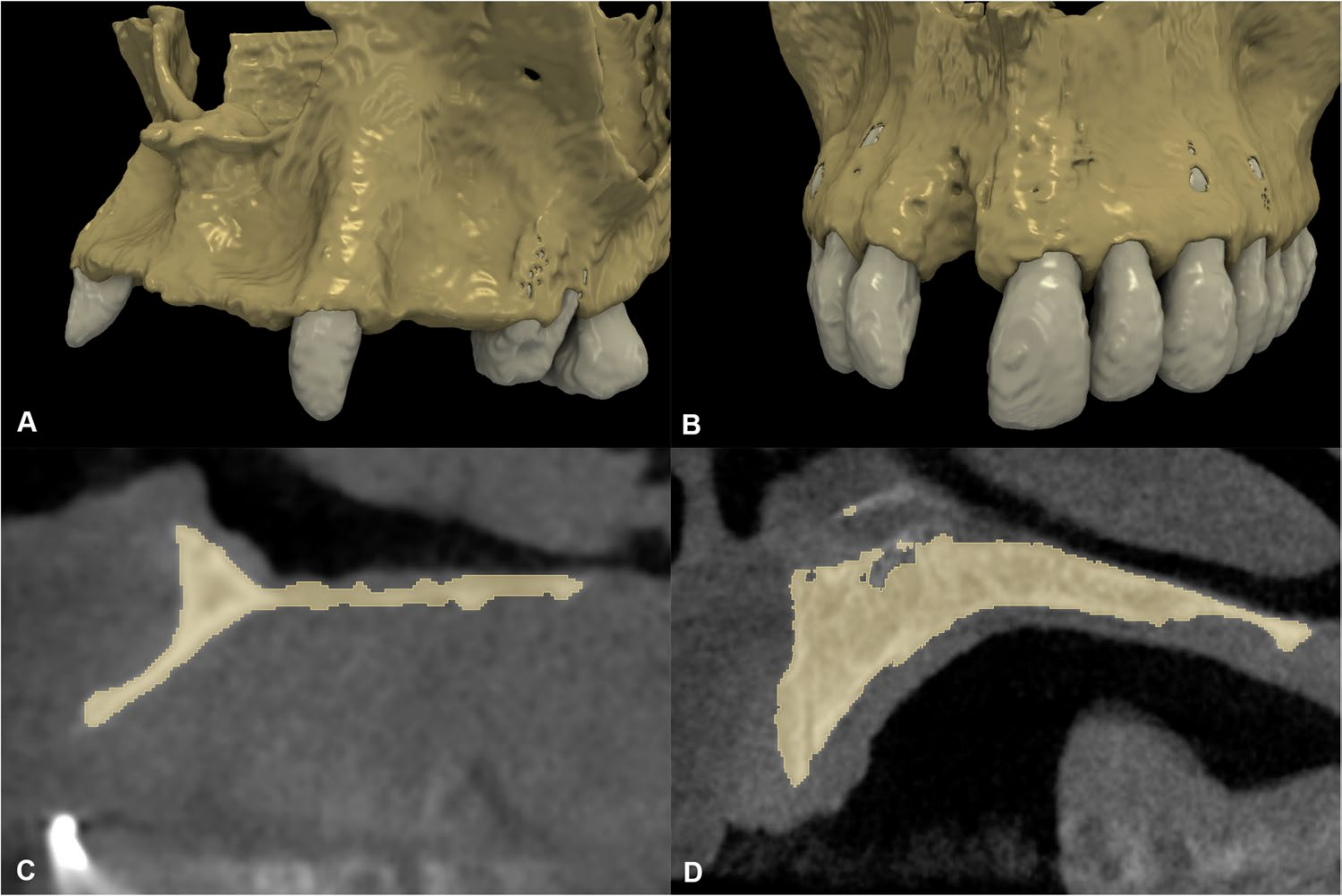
Baseline radiographic images. A 3D reconstruction with semi-automatic segmentation of an alveolar ridge defect at a multiple-tooth gap. B 3D reconstruction with semi-automatic segmentation of an alveolar ridge defect at a single-tooth gap. C Sagittal view of the baseline ridge dimensions at a multiple-tooth gap. D Sagittal view of the baseline ridge dimensions at a single-tooth gap
